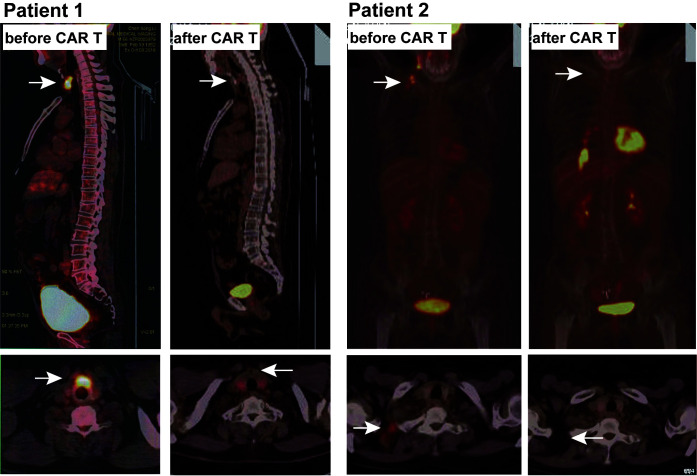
PET-CT images of two patients before and after CART treatment.
